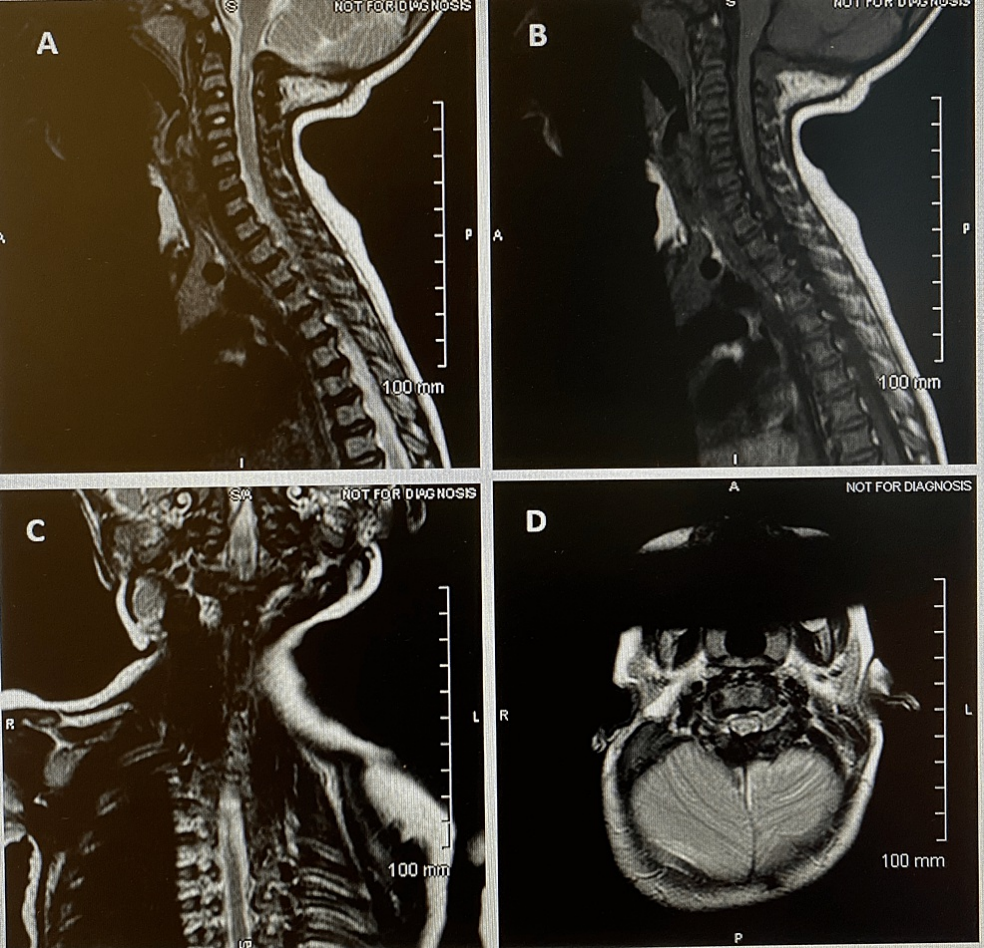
Post-operative MRI images Images A (sagittal T2), B (sagittal T1), C (coronal T2), and D (axial T2) - MRI images from 2017 February post-foramen magnum decompression surgery - improvement in foramen magnum stenosis with increased CSF space ventral to the cervicomedullary junction, axial and sagittal images. MRI, magnetic resonance imaging; CSF, cerebrospinal fluid
